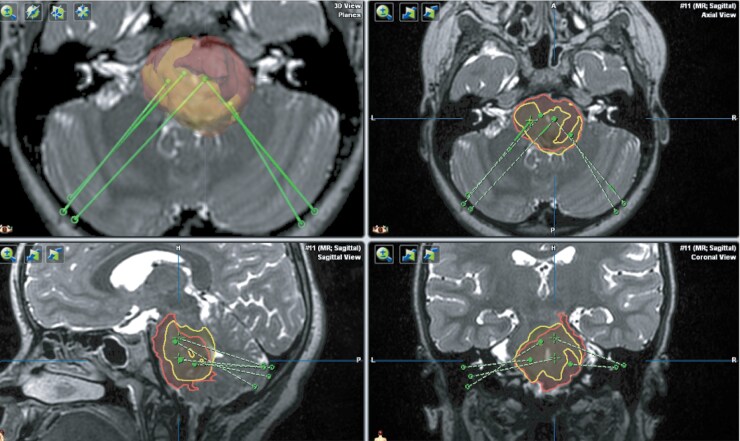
3D T2-weighted MRI overlay of baseline tumor volume and post-CED infusate distribution (PNOC009-4). Tumor volume at baseline (red) and total volume of infusate distribution (yellow) after 5 CED treatments.
